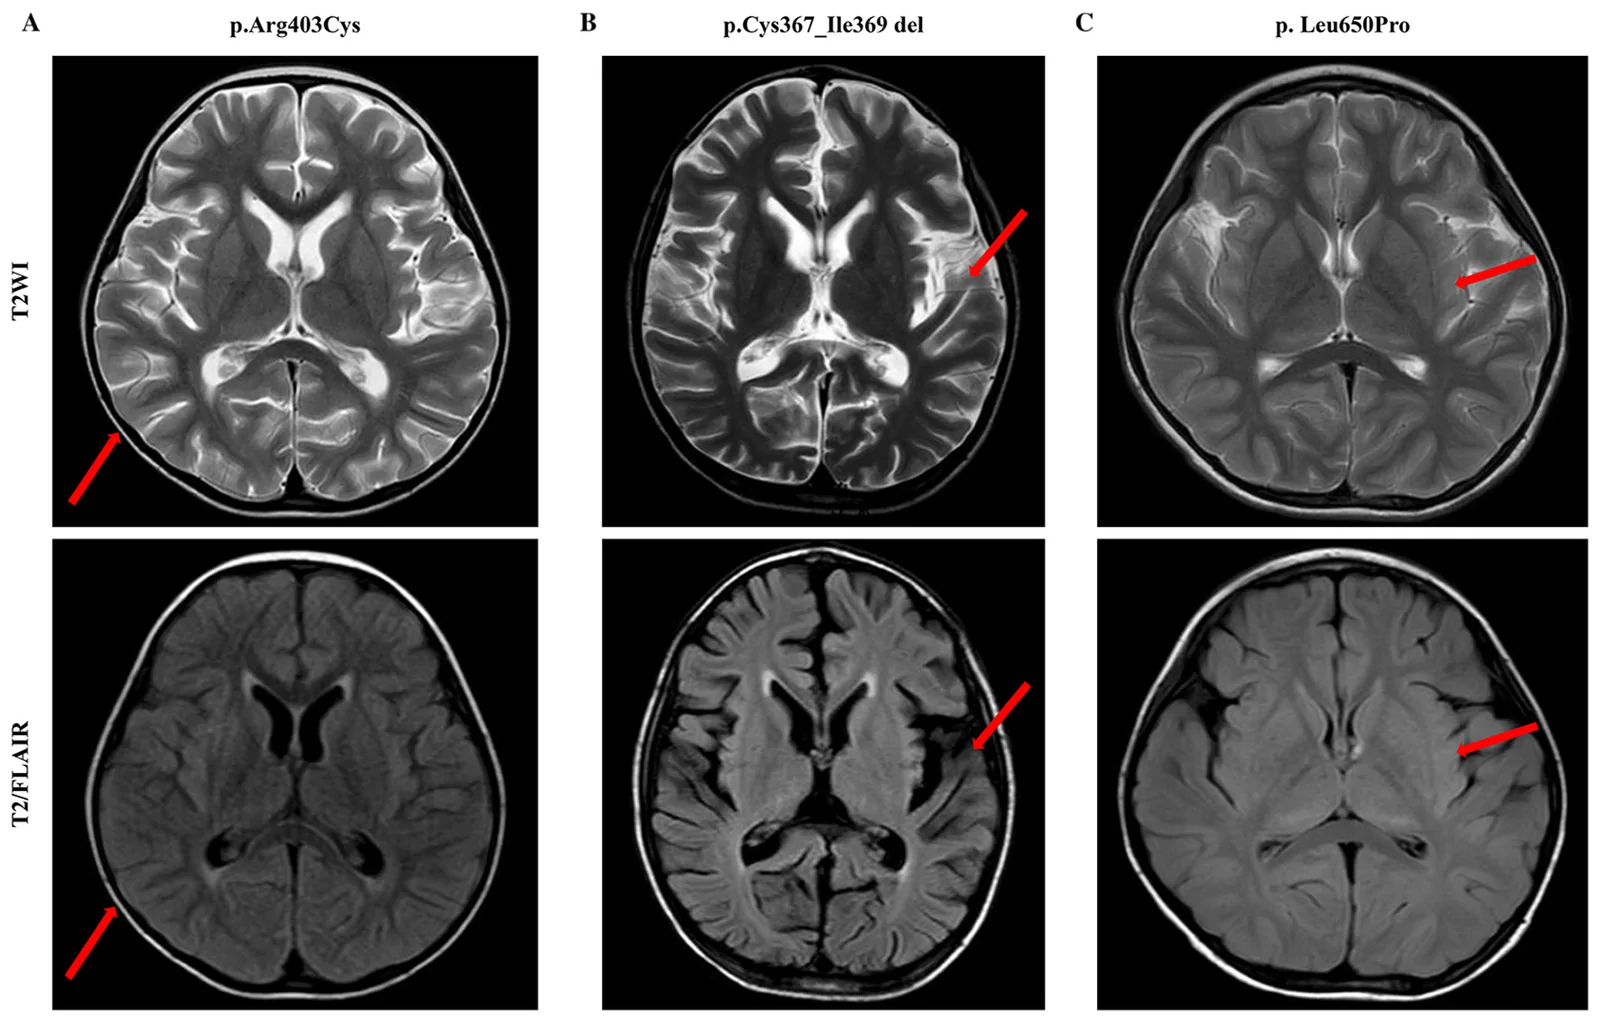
Representative axial brain MRI images of 3 patients with different *DNM1L* variants. (A) 4y7m, girl, 1 month since onset. The left column shows extensive swelling of the cerebral cortex in both hemispheres with hyperintensity on T2/FLAIR sequences. (B) 4y6m, boy, 1 year after onset. The middle column shows global cerebral atrophy and cerebral softening with gliosis. (C) 7y1m, boy, duration of illness: 2 weeks. The right column shows multiple T2 and T2/FLAIR hyperintensities in the bilateral cerebral cortex, along with symmetric lesions in the bilateral basal ganglia and thalamus. The red arrowhead indicates the representative area of the lesion. T2WI, T2-weighted image; T2/FLAIR, T2-Fluid-attenuated inversion-recovery sequency.
